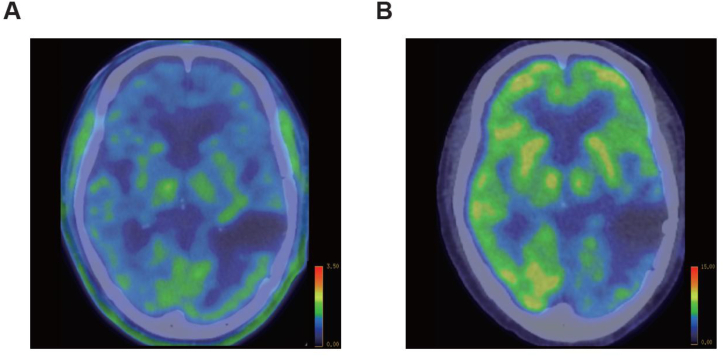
PET/CT fusion images. ^11^C-methionine PET image (A) and ^18^F-fluorodeoxyglucose PET image (B) at the patient's diagnosis of cerebral radiation necrosis (CRN). The standardized uptake value (SUV) is represented by the color scale.
